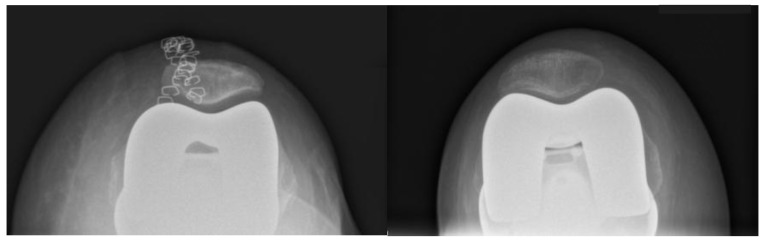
Example of an axial radiograph of the patella (Merchant view) used for measurements.
